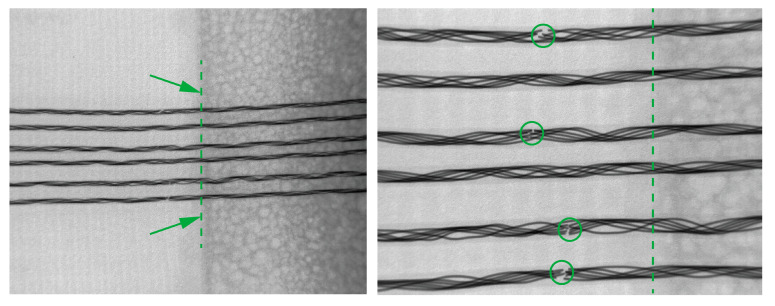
Photos of the broken yarn under x-ray. The yarn’s diameter is 0.6 mm and the pitch between the yarns is 1.27 mm. The arrows and circled areas highlight the breaking points and dashed lines highlight the soft-rigid transition areas.
